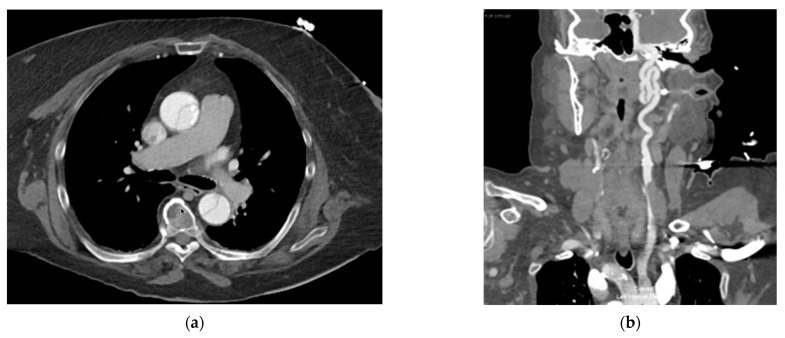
(a) Chest CT, demonstrating dissection near the transcatheter heart valve. (b) CTA head and neck, demonstrating near-complete occlusion of the right common carotid artery and complete occlusion of the right internal carotid artery.
